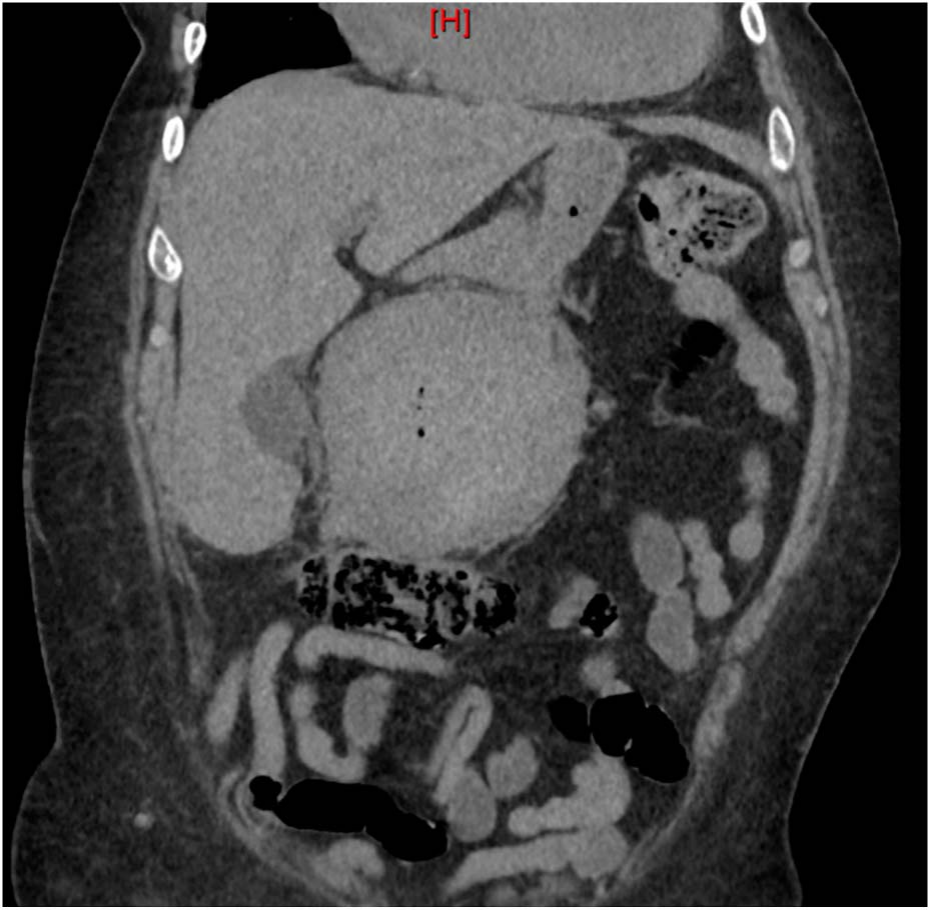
Computed tomography of the abdomen/pelvis without contrast: mild interval increased size of a hyperdense perigastric mass, recently biopsied. Previously favored to represent an exophytic gastric neoplasm given presence on previous chest computed tomography examination from 4 years earlier. However, recurrent lesser sac hematoma is a likely etiology, given appearance on recent endoscopy and imaging.
